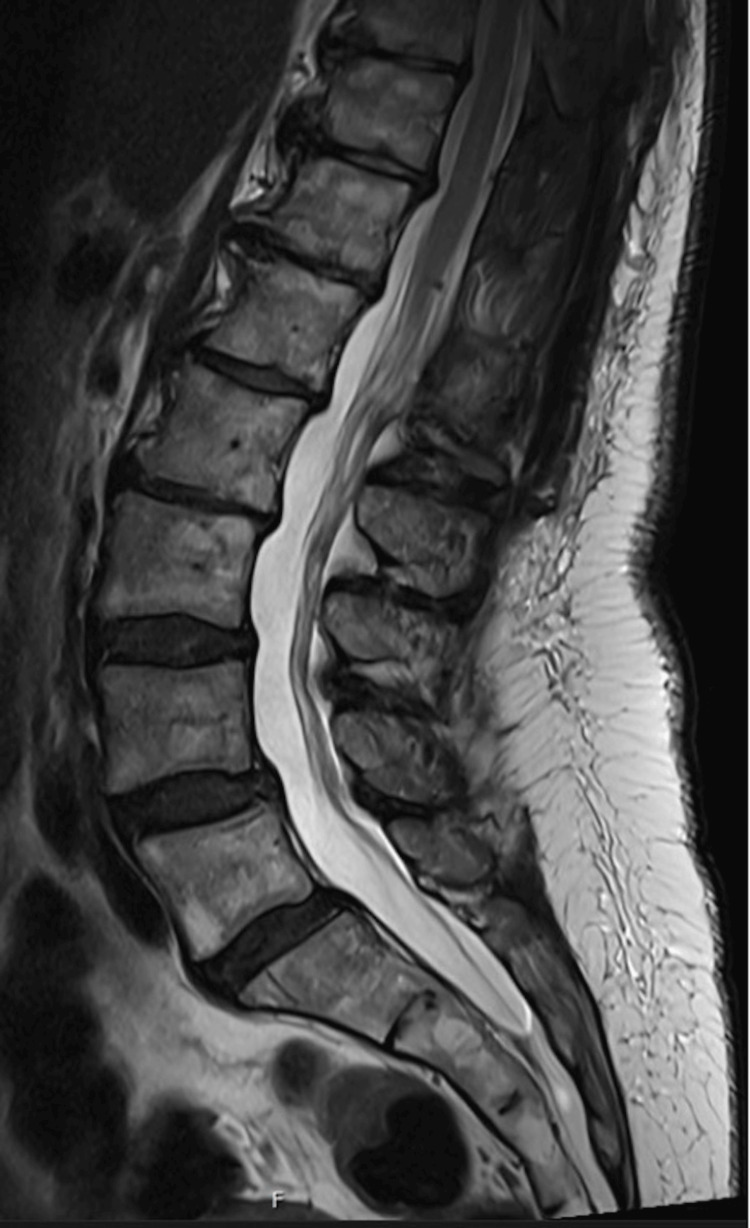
Postoperative T2-weighted sagittal MRI Postoperative T2-weighted sagittal MRI with resolved terminal syrinx and lack of artifact from the titanium fastener
